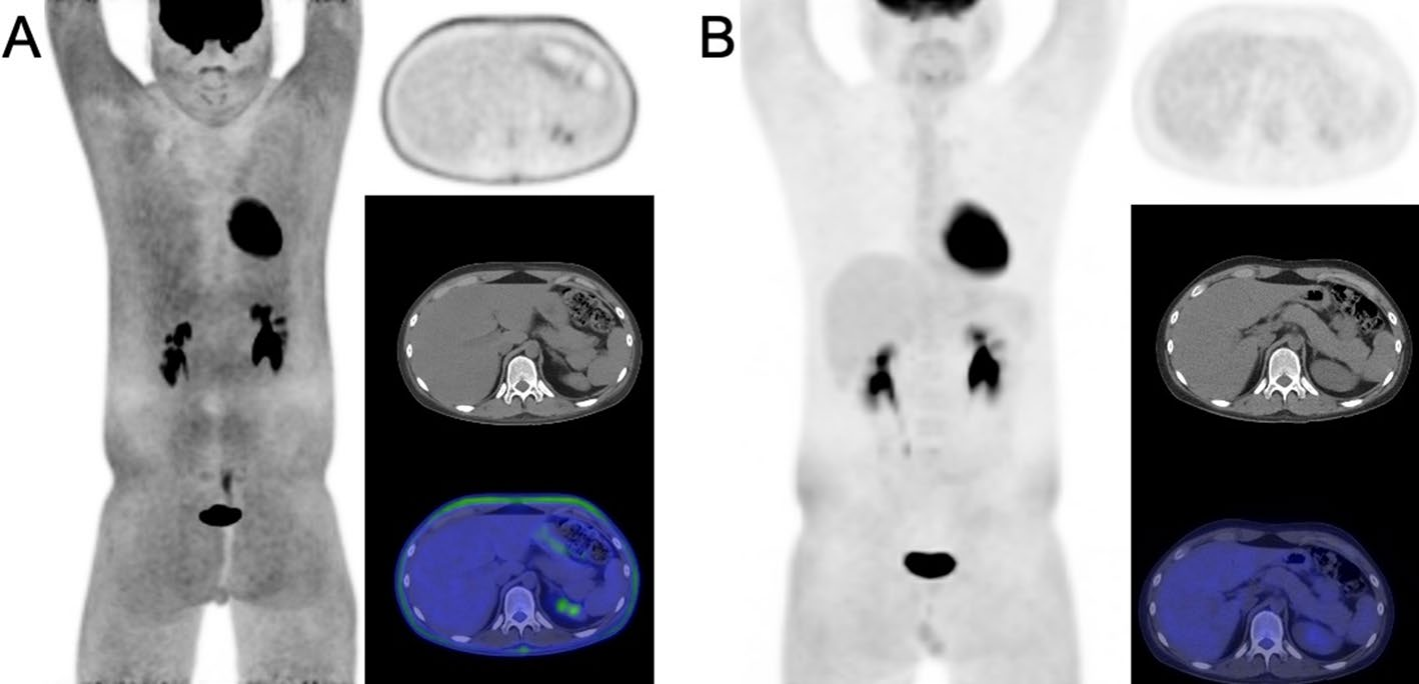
FDG-PET/CT images for restaging approximately one month after initial baseline imaging. Obtained two days after the patient finished a 29-day regimen of high-dose dexamethasone, the initial study (A) clearly shows diffuse FDG uptake in white adipose tissue, as seen on the maximum intensity projection (MIP), axial PET, and fused PET/CT imaging, limiting diagnostic utility. Repeat FDG-PET/CT performed 7 days later (9 days since last dose of dexamethasone) demonstrates that the increased interval since discontinuation of corticosteroids resulted in relative normalization of white fat activity in the upper extremities, chest, and abdomen, with mild persistent activity involving the white fat in the pelvis and proximal lower extremities (B)
